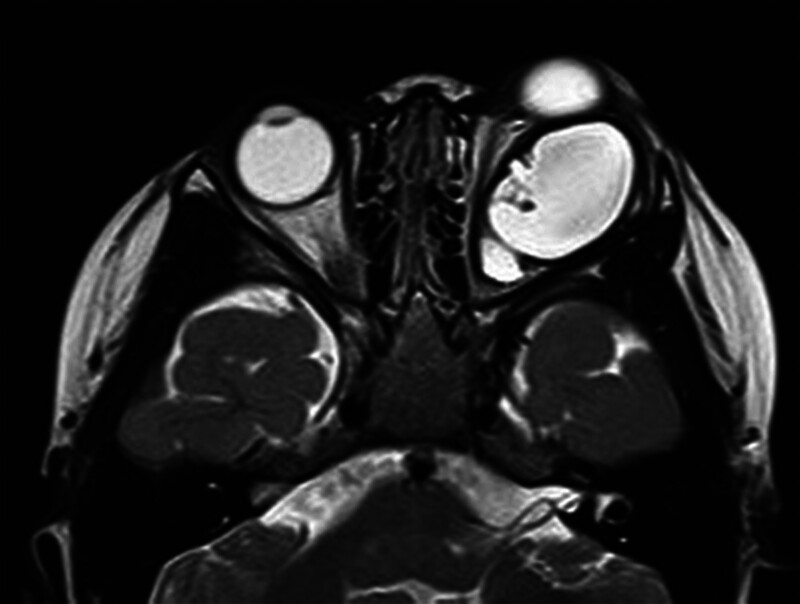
T2-weighted images (T_2_WI) highlighting hyperintense signals within the lesion. T_2_WI = T2-weighted images.
